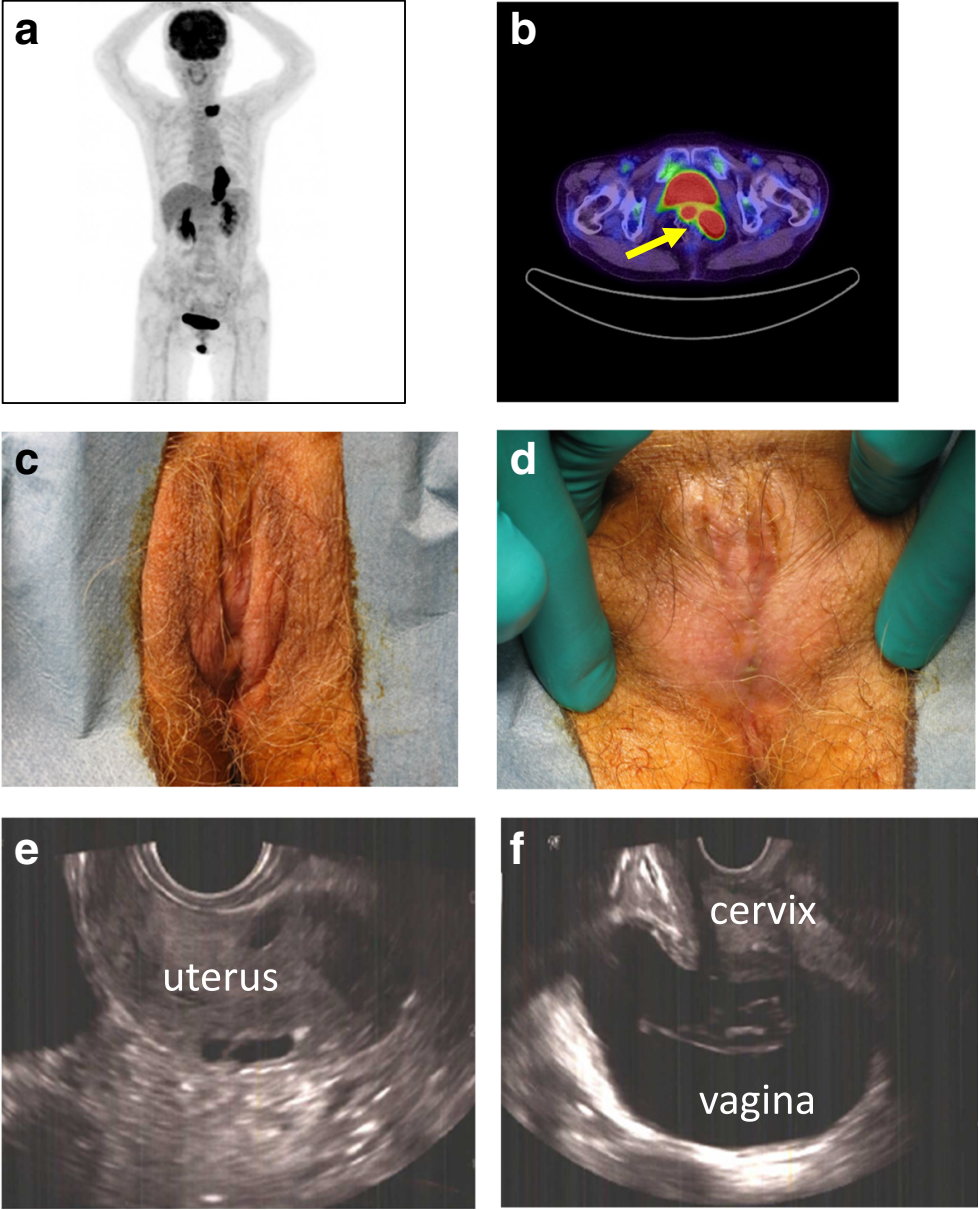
Examination findings at Out-patient Department. a Several hot spots were detected within the esophagus SUVMax 14.2), the left supraclavicular lymph node SUVMax 8.2), and the lesser curvature of the stomach SUVMax 5.5) in positron emission tomography scan. b A high accumulation, SUVMax 39.1, was found in the vagina and the cervix (*arrow*). c Inspection of the vulva. d The labia majora is completely fused and there is a pinhole opening in the midline. The vaginal introitus and urethral meatus are obliterated. e A transrectal ultrasound revealed the uterus was retroflexed and the endometrium was thin. f The vagina was dilated with fluid retention and a normal cervix was visualized
